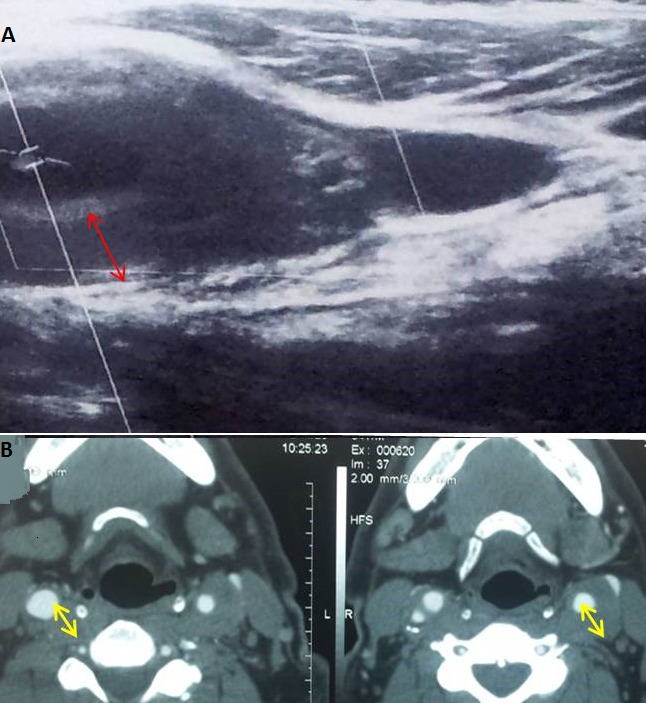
A) échographie Doppler des vaisseaux supra-aortiques montrant un épaississement de l'artère carotide commune (flèche rouge). B) angioscannar des vaisseaux supra-aortiques objectivant un épaississement étendu et fusiforme de l'artère carotide primitive gauche avec une sténose estimée à 60% de l'artère carotide interne droite (flèches jaunes)
